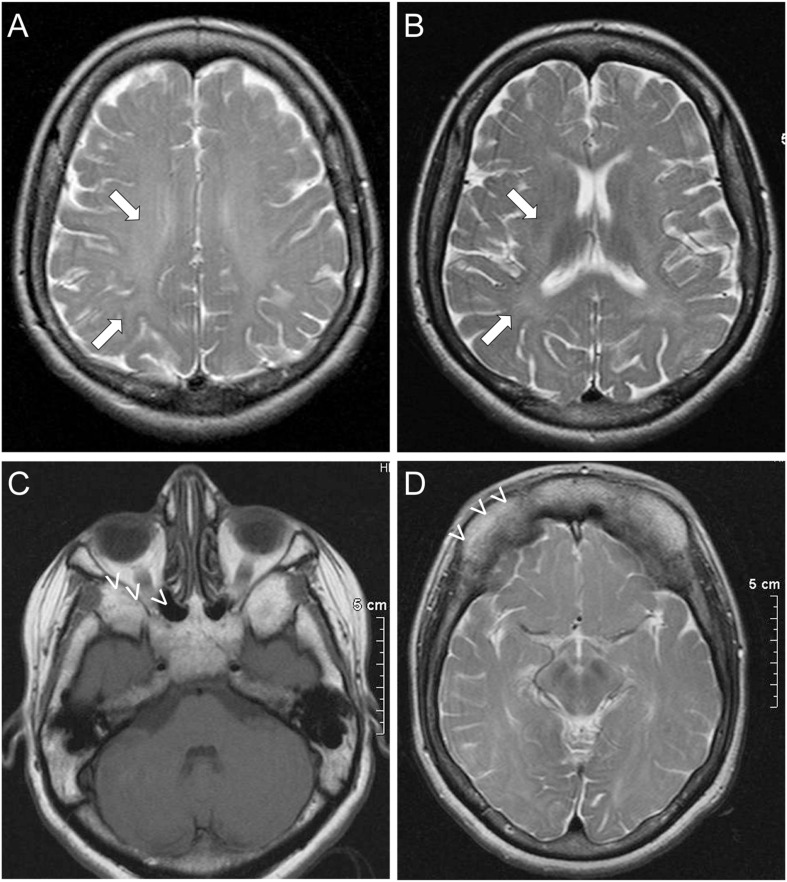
Imaging features of ODDD: Brain MRI of patient II shows subtle findings of leukodystrophy, with delayed myelination in the white matter (arrows) most prominently of the frontal and parietal lobes in the centrum semiovale (A) and toward the internal capsules (B). Dysmorphic features of ODDD including hypoplasia of the nasal and maxillary sinuses with small orbits (C) and complete aplasia of the frontal sinuses (D) are also demonstrated (open arrowheads).
